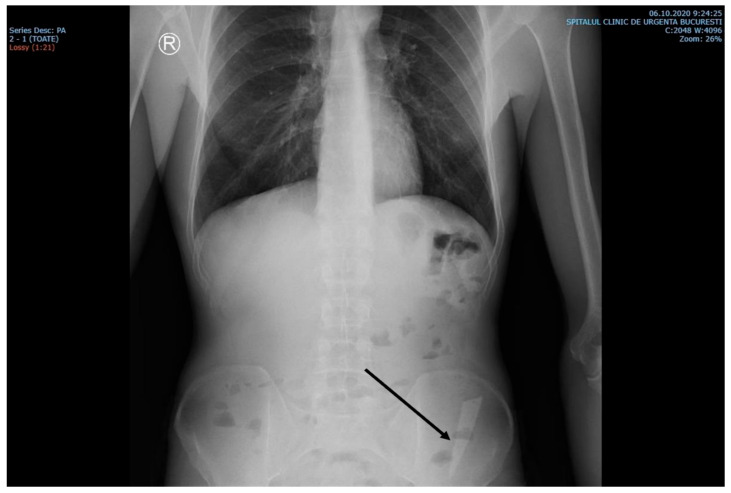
X-ray examination showed an elongated blade (arrow) in the iliac fossa, possibly in the sigmoid colon.
